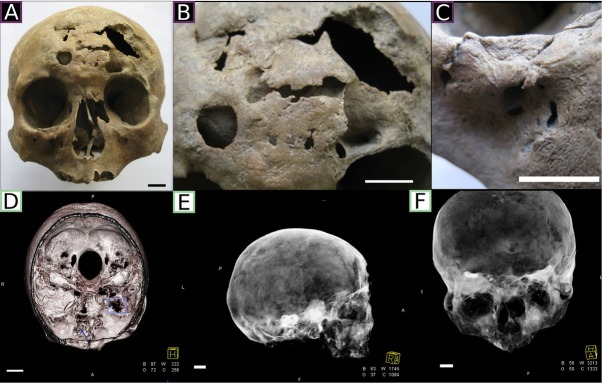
The skull 2000. A: Frontal view. B: Highly damaged frontal bone. C: Fracture in the right zygomaticomaxillary suture. D: Cross-horizontal-section of the skull in CT. Arrow and square indicate the inflammation on the base of the skull (anterior and middle cranial fossa) E: CT image of the right side of the skull. In the base of the vault lucencies are visible. F: CT image of the front of the skull. All damages and lesions of the skull have been thoroughly described in the results section. Scale bar=1cm.
